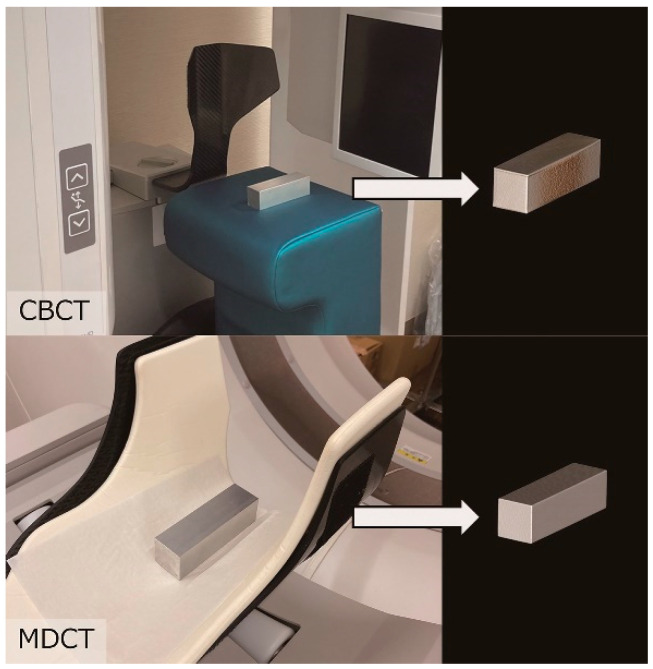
Validation of cone-beam computed tomography (CBCT) and multi-detector computed tomography (MDCT) measurement accuracy. Images show a known volume of aluminum blocks on each CT device. Three-dimensional CT images of these blocks were acquired using optimized experimental parameters.
